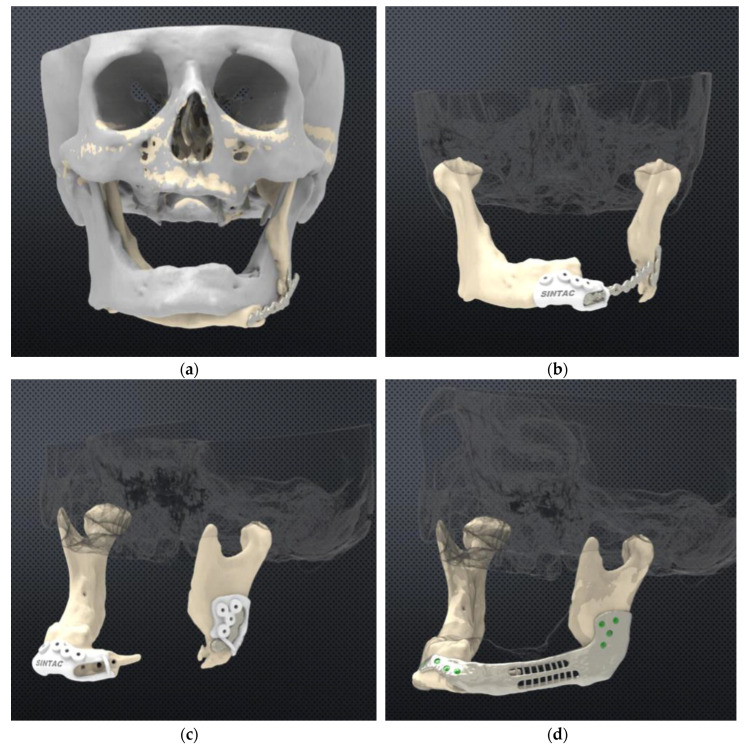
Computer-aided design of plate and drilling guides. (a) Superimposition of the native mandible (grey) and postresection (yellow) CT-scan data, showing mandibular displacement for plate rupture. (b) After mandibular segment 3d-repositioning; the design of the symphysis cutting and drilling guide, fixed on a fractured plate. (c) Design of ramus cutting and drilling guide. (d) Design of customised bridging mandibular prosthesis (CBMP).
